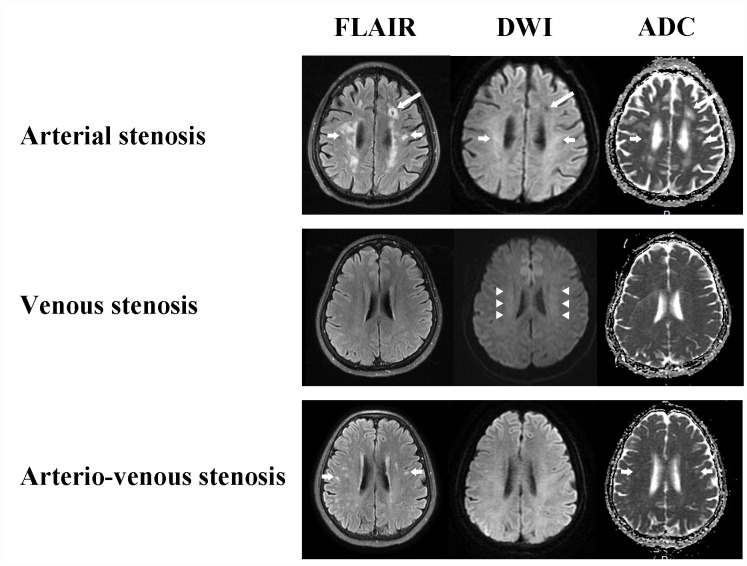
White matter changes of CAS, CVS and CAVS on MRI maps. Arterial stenosis always causes cavity (long arrows) and non-symmetrical multiple round, ovoid, patch and fused hyperintensity lesions with clear boundaries (short arrows). Venous stenosis conduces bilateral and symmetrical cloudy-like white matter hyperintensity surrounding ventricles and centrum semiovale (arrow heads). Arterio-venous stenosis also has non-symmetrical focal white matter lesions (short arrows).
